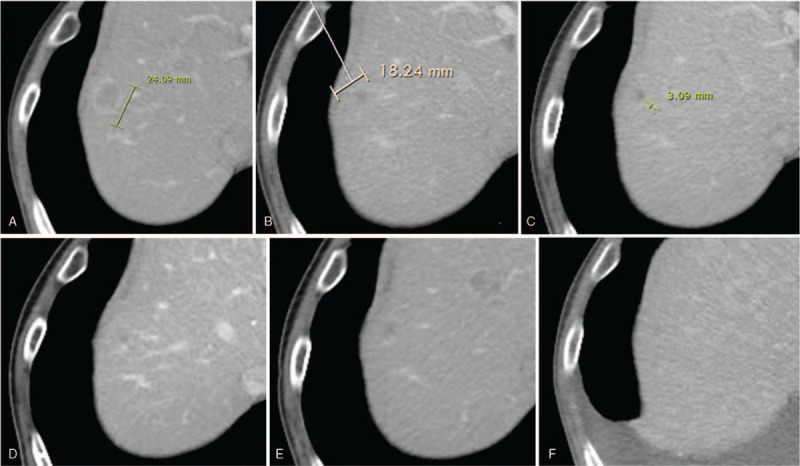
Computed tomography images show the liver metastasis. (A) At the start of chemotherapy; (B) after 2 cycles; (C) after 4 cycles; (D) after 6 cycles; (E) after 9 cycles; and (F) after 12 cycles of chemotherapy. While the liver metastatic mass expanded to 24 mm in diameter at beginning of chemotherapy, the mass reduced to subcentimeter sized (3 mm) after 4 cycles of chemotherapy.
